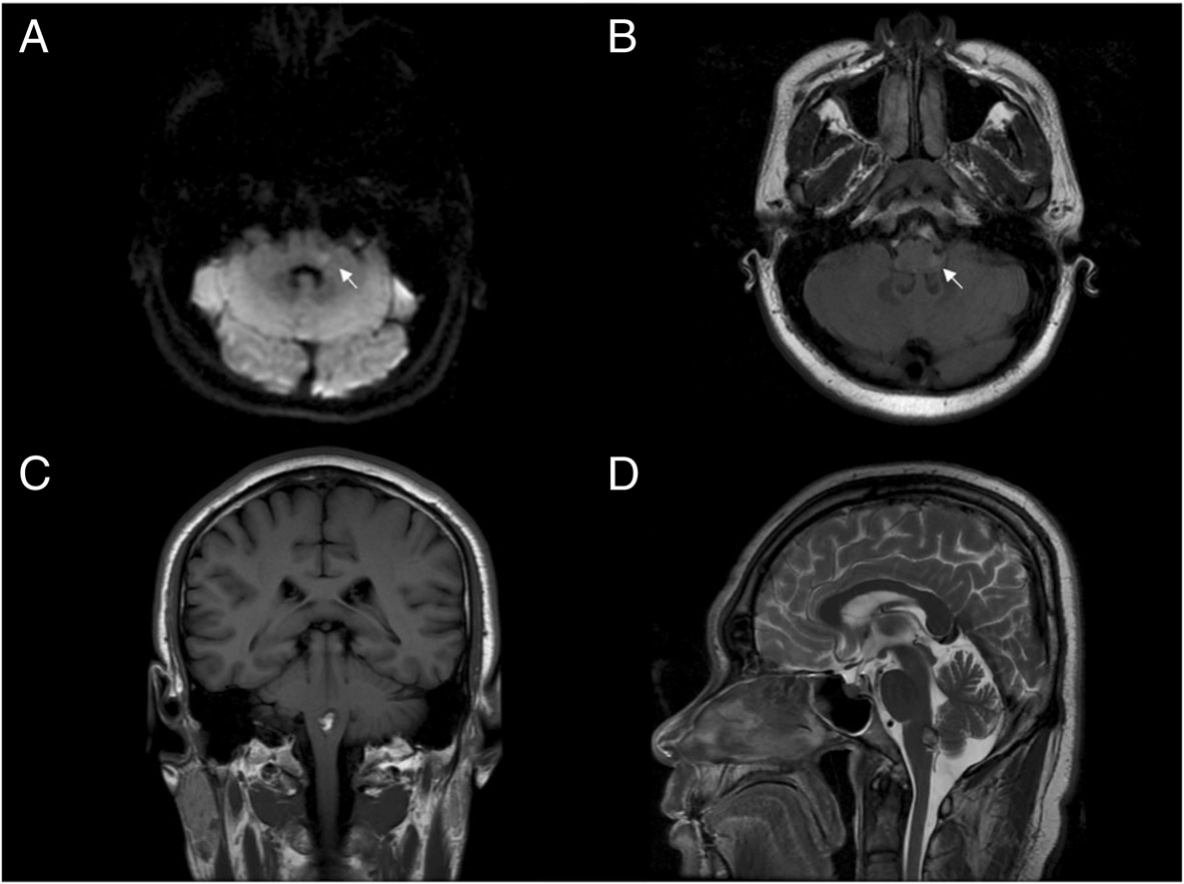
Two cases of unremarkable focal neurological or nystagmus findings but marked SVV deviations. a, b A young man (30–35 years) complaining of dizziness only had equivocal facial numbness and end-gaze nystagmus. However, the bucket test revealed an SVV deviation of 11 degrees to the left; MRI showed a tiny infarct in the left lateral medulla (arrow). c, d An older man (50–55 years) with 10 days of dizziness but no focal neurological signs or nystagmus: His bucket test showed an SVV deviation of 5 degrees to the left; MRI showed a cavernous hemangioma in the dorsal medulla
